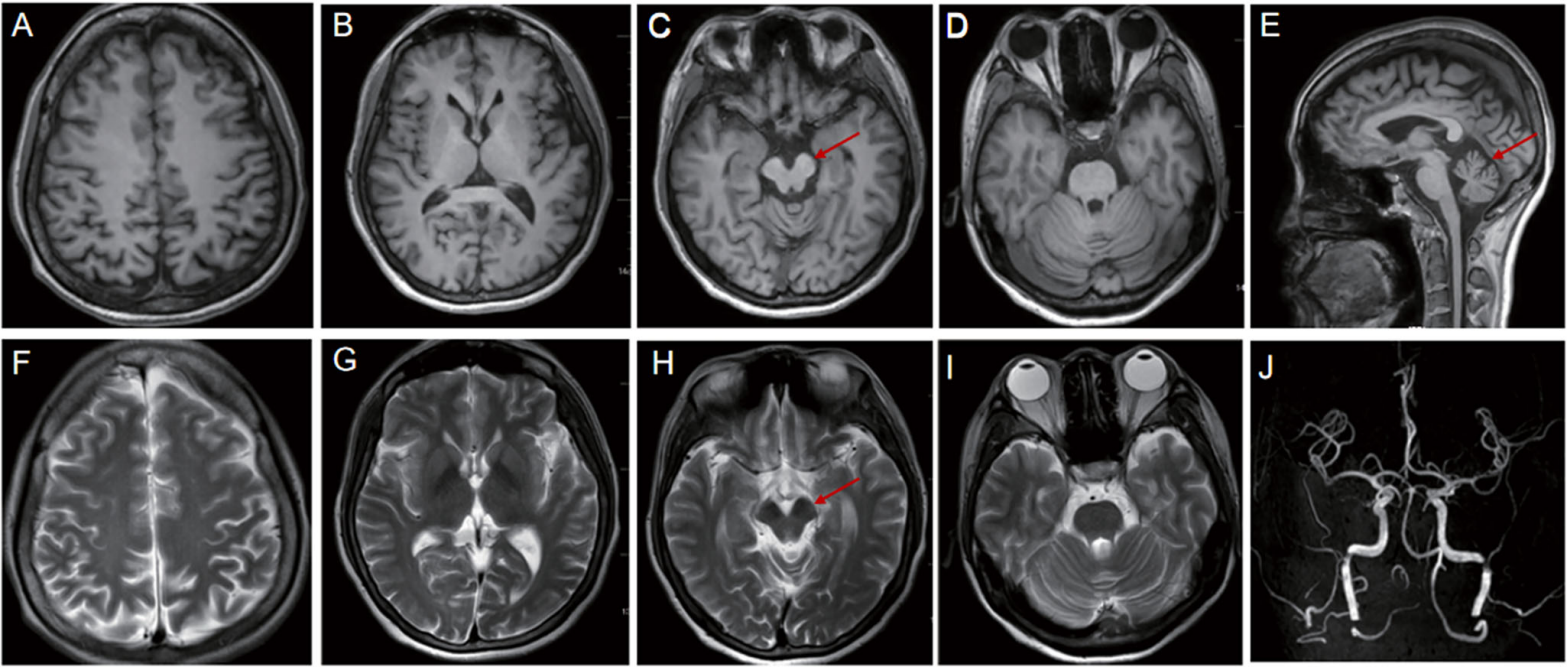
The brain MRI findings of the proband. (A) Transverse plane T1‐weighted (T1W) image at the level of the centrum semiovale. (B) Transverse plane T1W image at the level of the basal ganglia. (C) Transverse plane T1W image at the level of the midbrain. The arrow indicates midbrain atrophy. (D) Transverse plane T1W image at the level of the pons. (E) Sagittal plane T1W image. Arrow indicates cerebellar atrophy. (F) Transverse plane T2‐weighted (T2W) image at the level of the centrum semiovale. (G) Transverse plane T2W image at the level of the basal ganglia. (H) Transverse plane T2W image at the level of the midbrain. Arrow indicates midbrain atrophy. (I) Transverse plane T2W image at the level of the pons. (J) Magnetic resonance angiography (MRA) showing no significant abnormalities.
